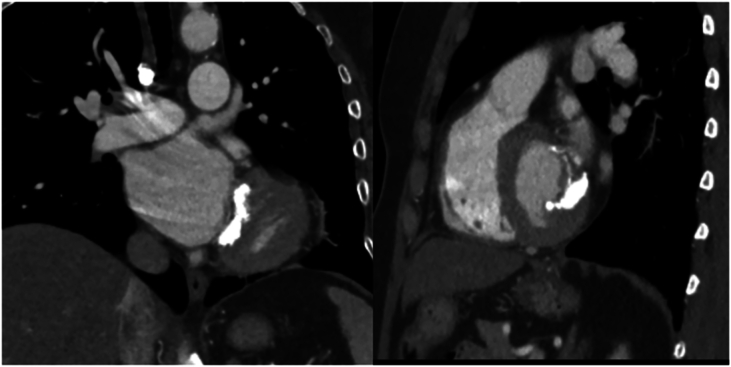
Computed tomography images of the chest demonstrating bioprosthetic mitral valve with dense mitral annular calcification.
